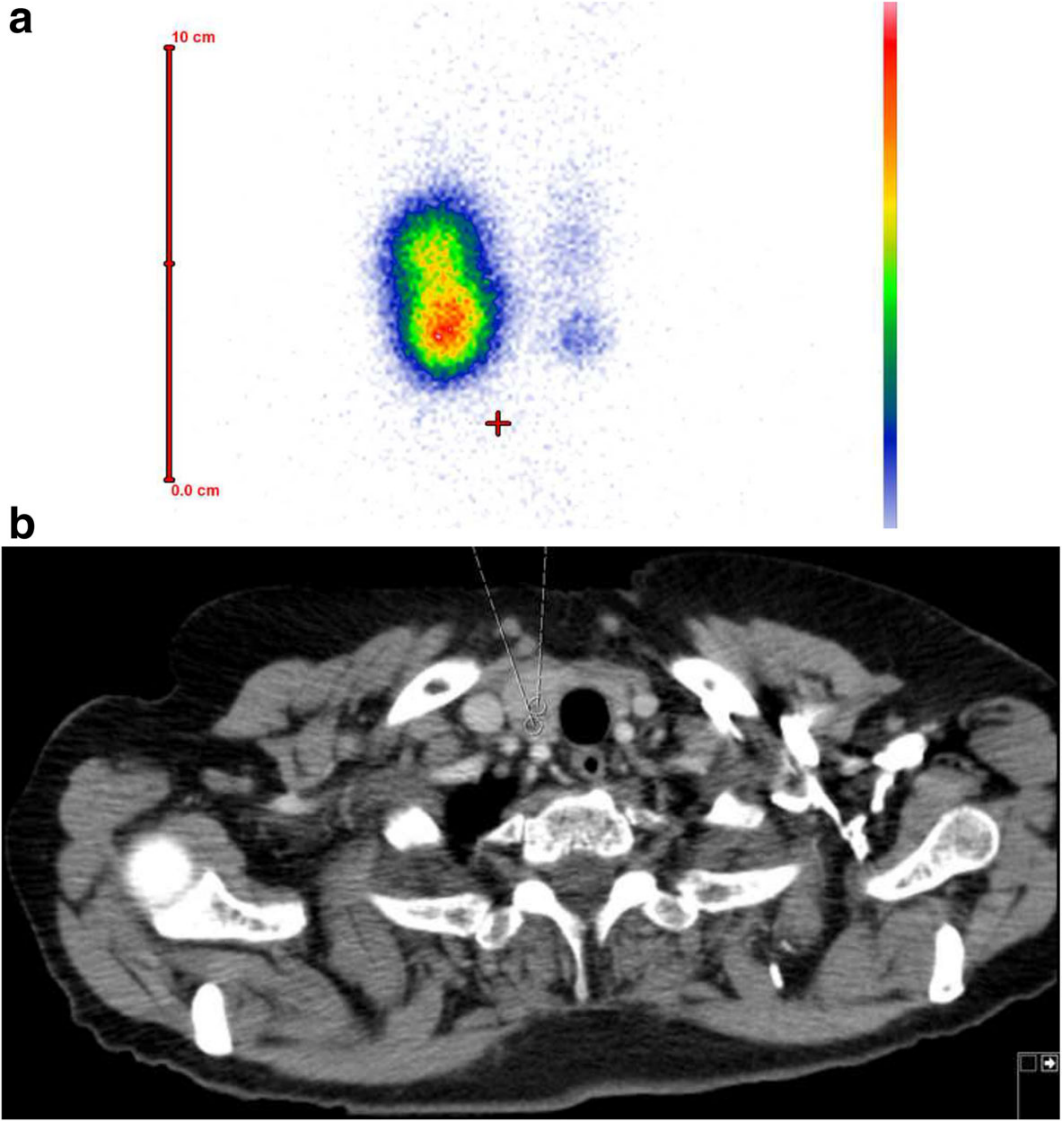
a, b TS shows an area with increased uptake in the right lobe of the thyroid caudal which is consistent with a hot nodule The chest CT depicts a nodule in the right lobe of the thyroid. Abbreviations: TS, Thyroid scintigraphy; CT, computed tomography
